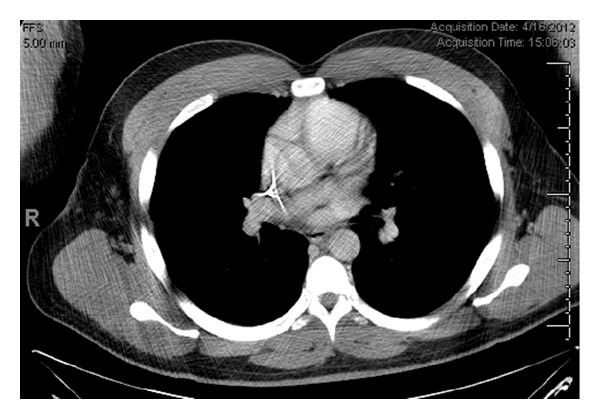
CT of abdomen in 30-year-old male with history of gallstone pancreatitis and pseudocyst with acute onset of abdominal pain.
